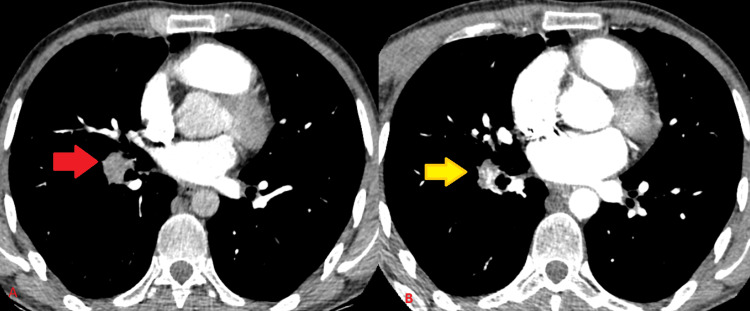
Treatment response evaluation with CT chest Compared to the sixth-month imaging (A-red arrow), reduction in pulmonary embolism and aneurysmatic structures (b-yellow arrow) led to the cessation of treatment in the ninth month CT: computed tomography
